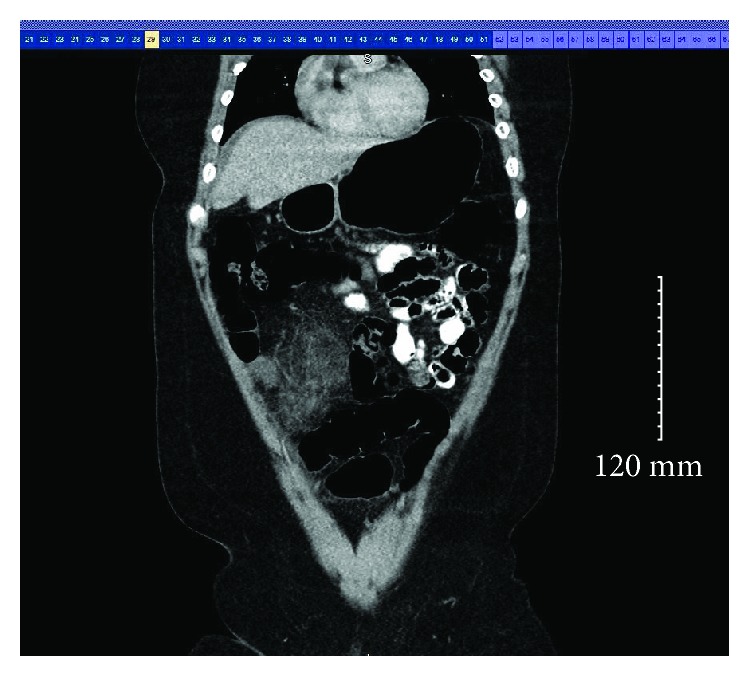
Coronal CT having signs of omental infarction.
